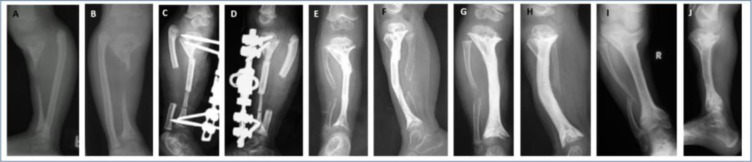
A 2-year-old girl presented with severe osteomyelitis and an exposed sequestrum. The infection was quiescent at presentation. Radiographs showed a short deformed leg, a large bone defect of the tibia and early physeal closure (a, b). Amputation was advised but was refused. A segment of the ipsilateral fibula was inserted between the trimmed bone ends, with concurrent axis correction and external fixation (c, d). At 13 weeks, the graft is integrating and remodelling (e, f). Radiographs at 14 months showed a short, sclerotic tibia with moderate procurvatum (g, h). An orthoprosthesis was prescribed. Ten years later, she had no infection relapse, just moderate knee and ankle pain with a severe LLD (15 cm). She walked normally without aids or a brace. The radiographs showed a short tibia with varus deformity due to medial tibial plateau depression (i, j).
